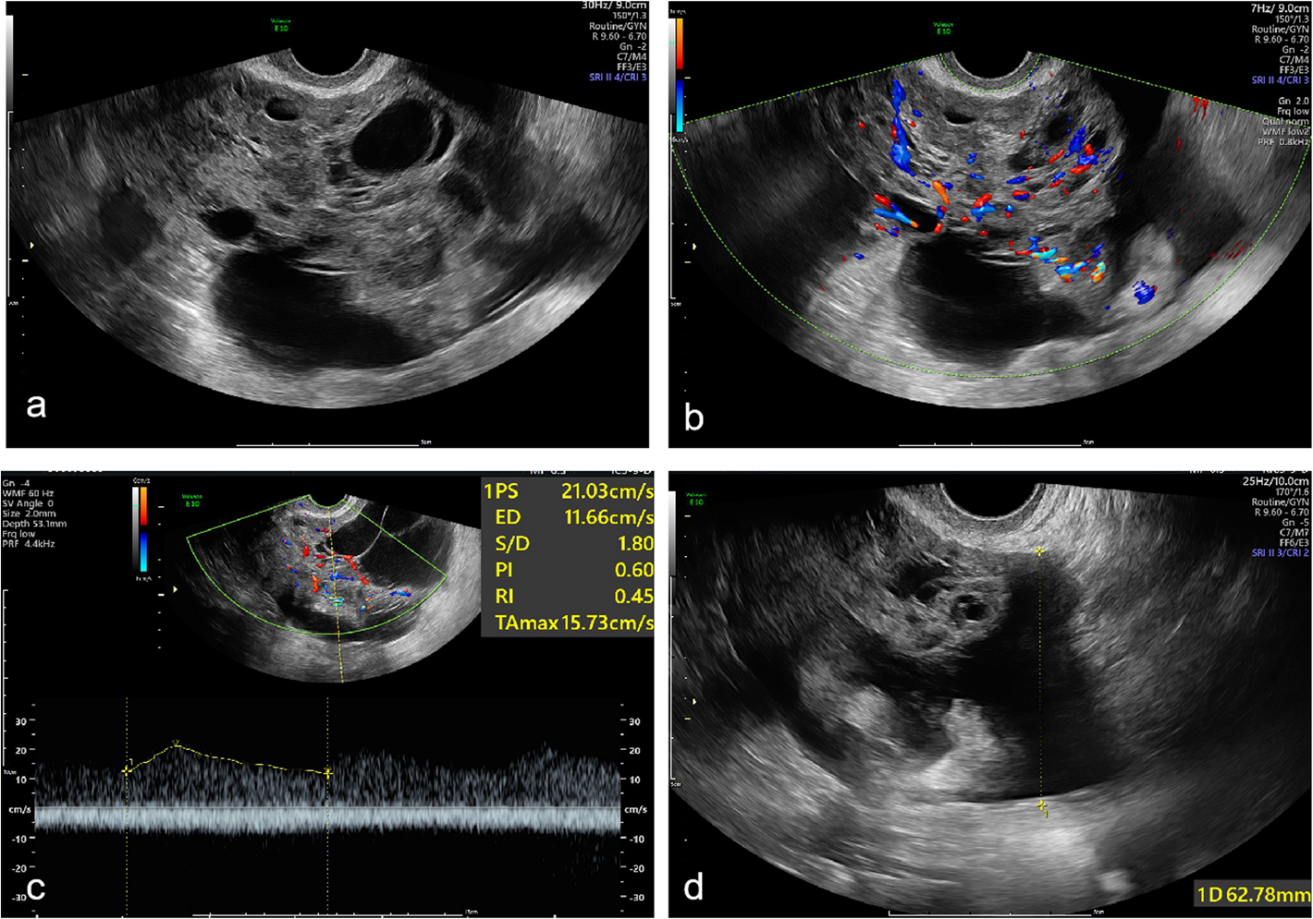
A 63-year-old woman suffered from OGCT. a a cystic-solid mass was detected in the right ovary by two-dimension ultrasound examination; b the Color Doppler showed the mass with abundant doppler flow signal; c the Spectrum Doppler showed the mass with low velocity blood flow; d ultrasound detected ascites in the pelvis
